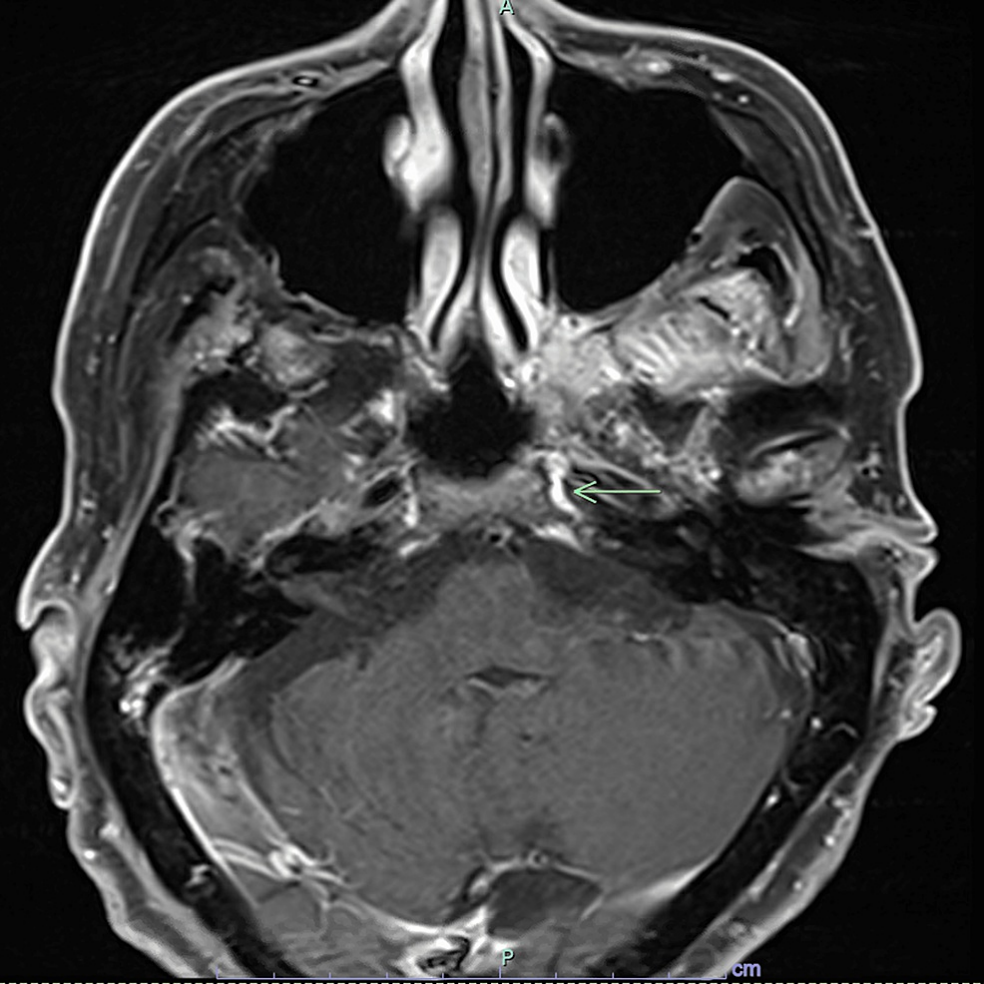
MRI of the brain revealed an enhancing lesion of the right intracranial prechiasmatic optic nerve
